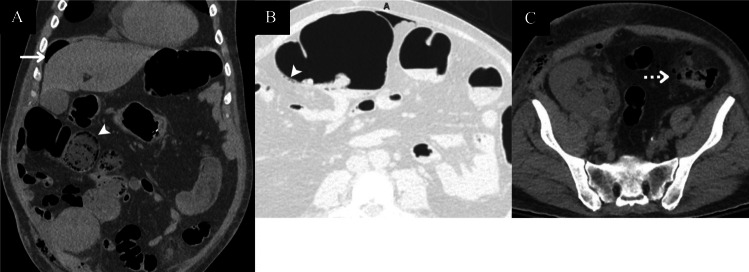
52-year-old patient with acute perforated sigmoid diverticulitis. Coronal CT image A shows PI of the cecum (arrowhead) and pneumoperitoneum (solid arrow). Axial CT image B shows PI of the cecum (arrowhead). Axial CT image C shows perforated sigmoid diverticulitis (dashed arrow). This patient underwent an exploratory laparotomy which showed a dilated cecum with no signs of ischemia
